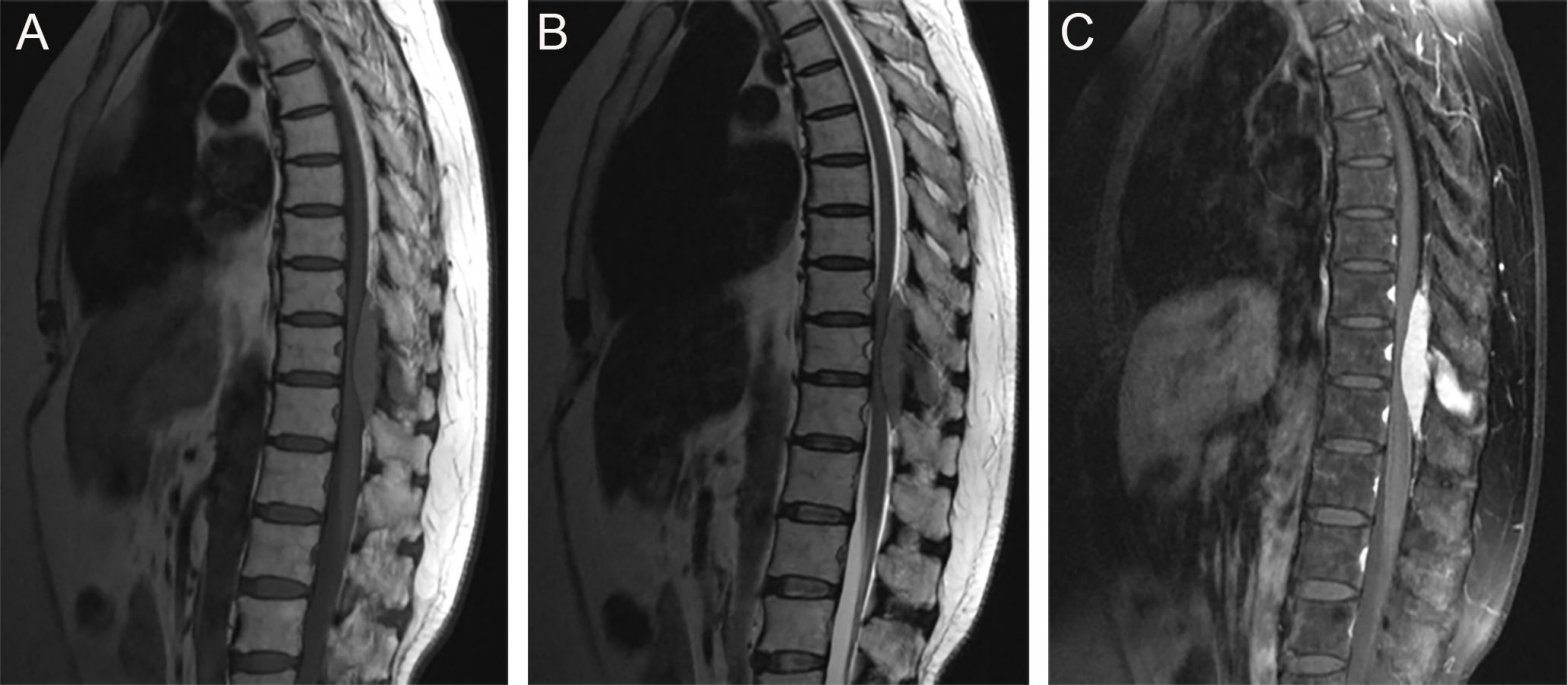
Sagittal MRI images showed a well-circumscribed extramedullary lesion with the destruction of adjacent bone, ranging from T10 to T12. (A) T1WI; (B) T2WI; (C) postcontrast T1WI.
